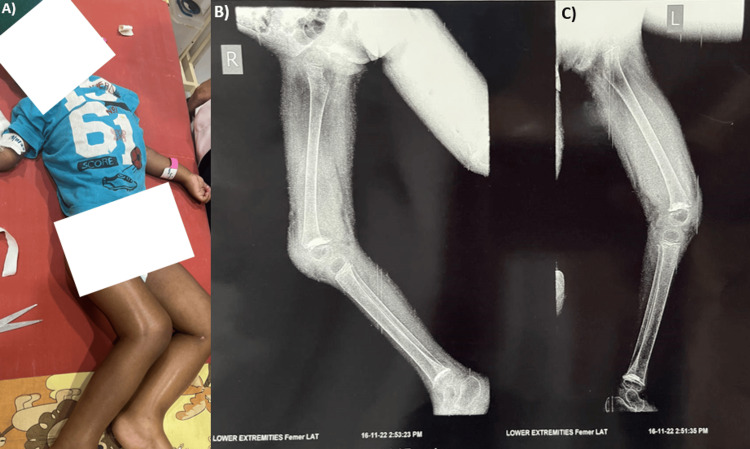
Clinical and radiology images of the patient A) A clinical picture of the patient presenting severe acute malnutrition; B) A lateral X-ray image of the right lower limb, confirming features of scurvy; C) A lateral X-ray image of the left lower limb, confirming features of scurvy
